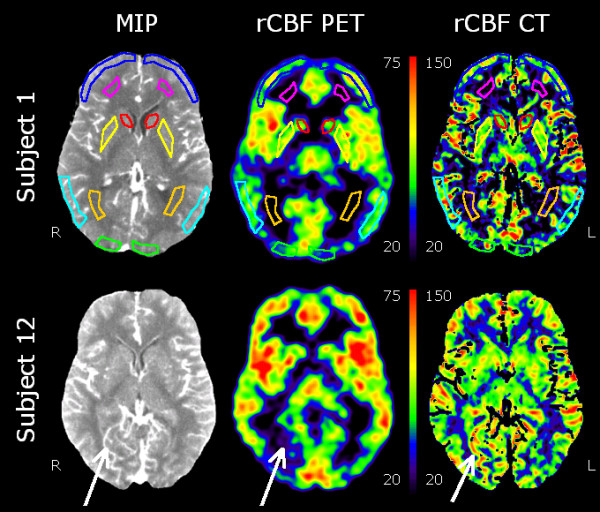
Co-registered transaxial slices through the level of the basal ganglia. Co-registered transaxial slices showing MIP from perfusion CT integrated over 40 s (left), the rCBF images using PET (centre), and perfusion CT (right) quantified in millilitre per minute per 100 g. For a better comparison, the rCBF PET and rCBF CT images are displayed in different scales. The top row shows the location and configuration of ROIs in the frontal cortex (dark blue), parietal cortex (light blue), occipital cortex (green), caudate nucleus (red), putamen (yellow), frontal white matter (purple), and occipital white matter (orange). High-intensity areas in the MIP images represent larger vascular volumes around the vessels of the cortex and insula, the choroid plexus, and the sinuses. These are partly, but not completely, removed by masking the rCBF CT images. This discrepancy between the two techniques is evident around a right-sided occipital vein that is visualised in rCBF CT, but not in rCBF PET (white arrow, bottom row).
